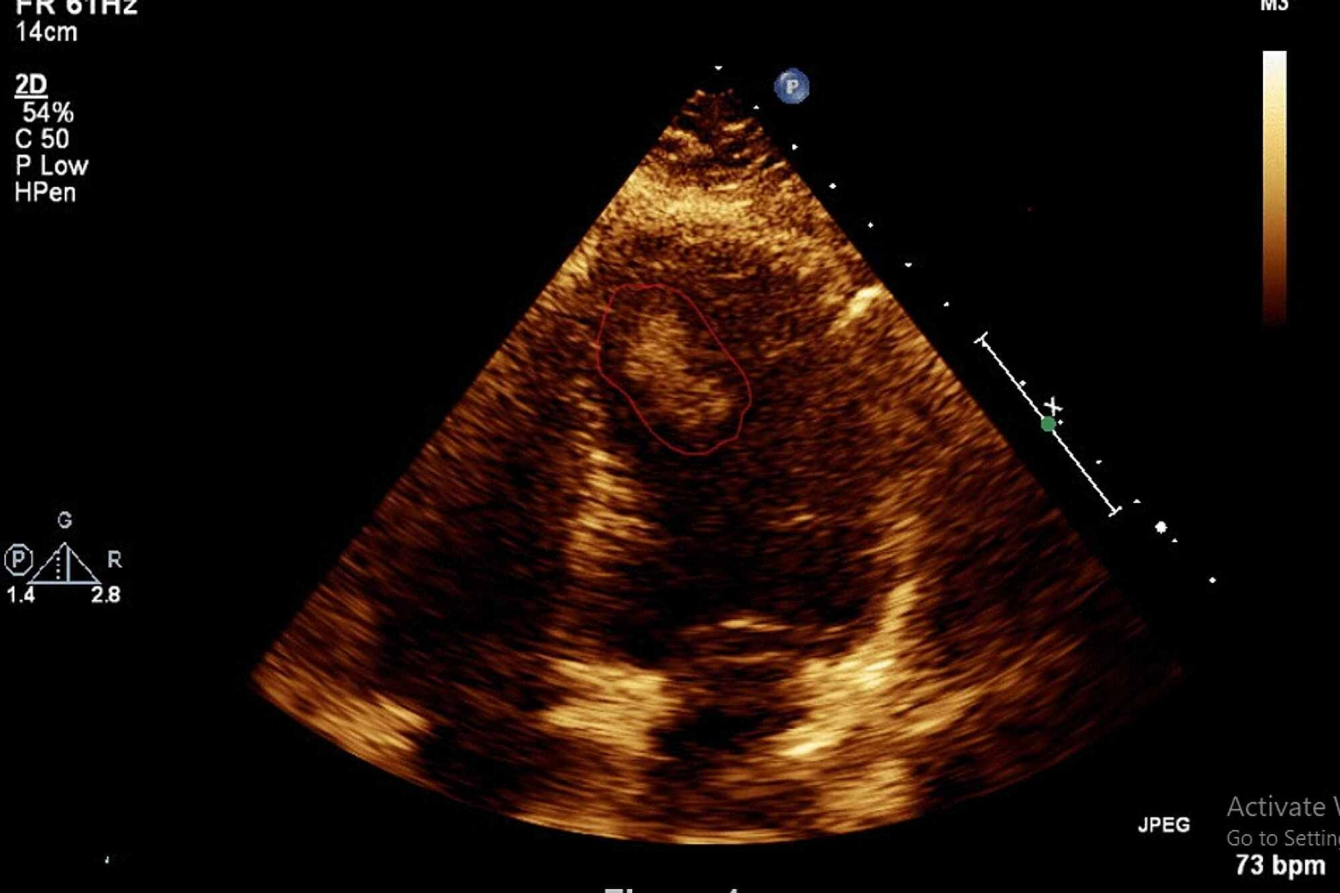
Transthoracic Echocardiography showing apical/septal mass in the LV (apical/four-chamber view)
